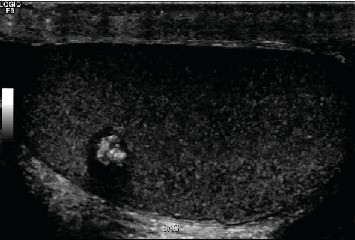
Ultrasound image showing 9 mm right testicular calcified lesion.
